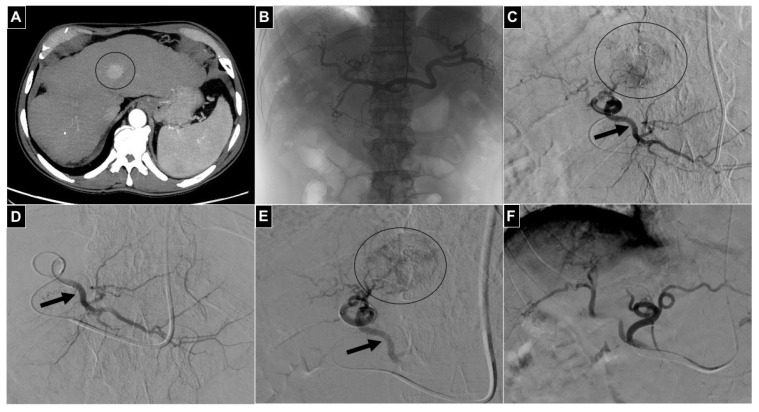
A 61-year-old male with multifocal HCC; treatment with DSM-TACE was selected. (A) A 3 cm nodule in segment IVa (black circle) is evident in the CT scan in the arterial phase. (B) Selective coeliac trunk arteriography is acquired via a 5 Fr diagnostic catheter positioned via radial access, and normal anatomy is appreciable. (C) Left hepatic artery is selected with a 2.4 Fr microcatheter and DSA is acquired; the HCC nodule (black circle) is refurnished by small branches not suitable for microcatheter selection. (D) Flow diversion by temporary embolization of segmental branches II-III is performed by advancing the microcatheter tip (black arrow). (E) After Embocept-S^®^ particle injection, stop flow into the segmental branches II-III is obtained (black arrow) and arterial flow is solely directed to the HCC nodule (black circle); the microcatheter tip is retrieved proximally and DSM-TACE is performed. (F) Final DSA control showing no more nodule opacification in segment IVa.
